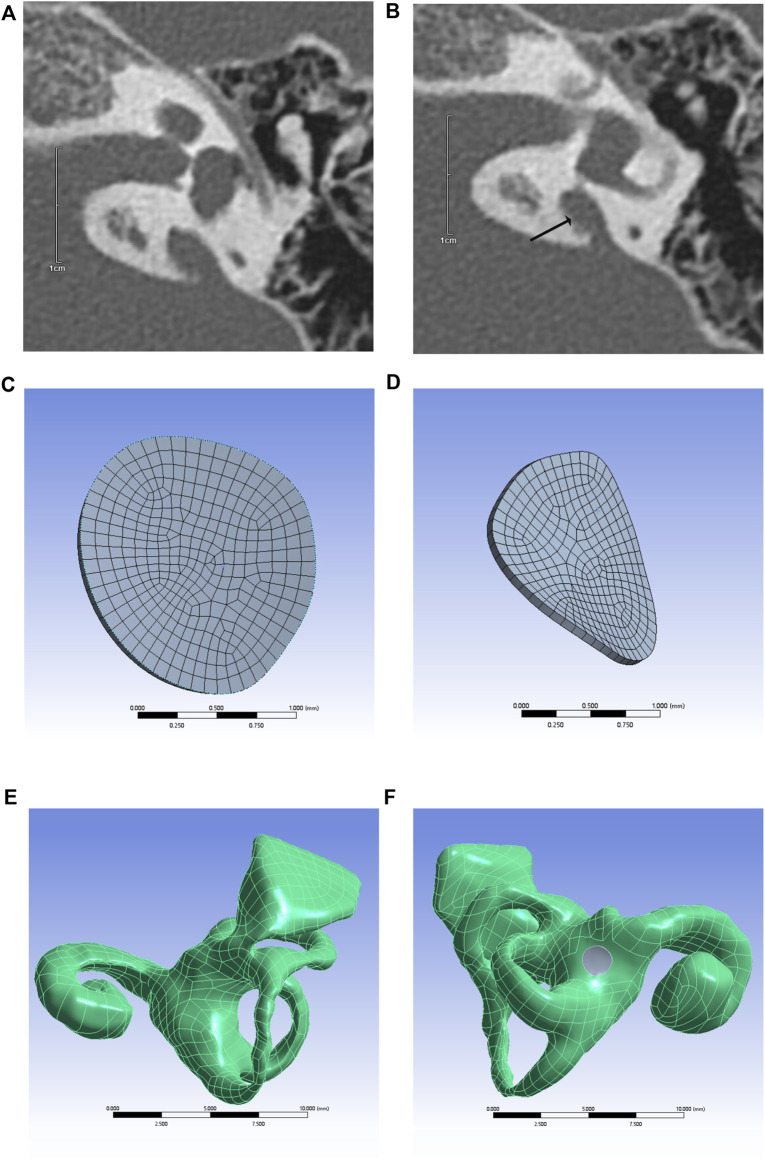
CT image of temporal bone with enlarged VA (arrows) (A,B), round window membrane model (C,D), 3D model of the inner ear (E), and inner ear model containing round window membrane model (round window membrane model is gray) (F).
